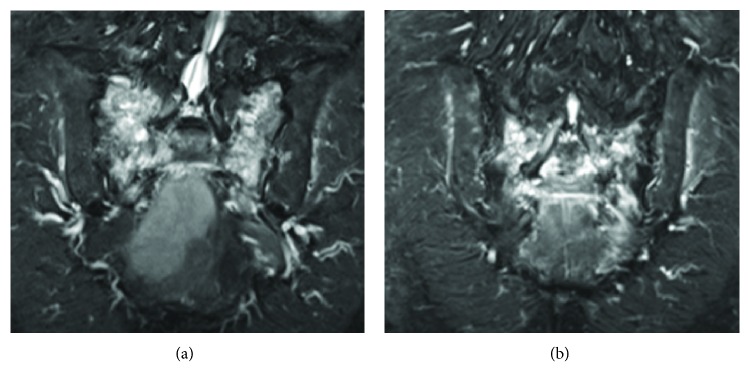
Lumbosacral magnetic resonance imaging with short inversion time inversion recovery (STIR) sequence shows an H-shaped sacrum fracture. Bilateral vertical fractures of the lateral mass of the sacrum and horizontal fracture at the level of the S2 neural foramina are visible.
